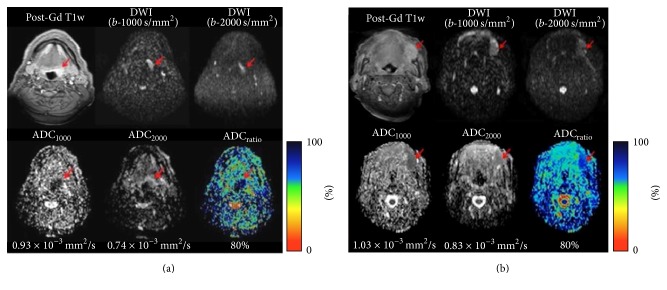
Representative MR images of recurrent tumors. (a) Patient number 12 (M, 68 yo) treated with chemoradiotherapy for a squamous cell carcinoma of the oropharynx. Postcontrast T1-weighted sequence shows an enhanced thickening of the left wall of oropharynx. (b) Patient number 20 (F, 74 yo) after surgery and radiotherapy for a squamous cell carcinoma of the tongue. Postcontrast T1-weighted sequence demonstrates an enhanced exophytic lesion of the left margin of the tongue. Recurrent tumors are characterized by high signal intensity on DWI at standard and high* b*-values and low signal intensity on the corresponding ADC maps. Mean ADC_1000_, ADC_2000_, and ADC_ratio_ were 0.93 × 10^−3^ mm^2^/s, 0.74 × 10^−3^ mm^2^/s, and 80% (a) and 1.03 × 10^−3^ mm^2^/s, 0.83 × 10^−3^ mm^2^/s, and 80% (b).
